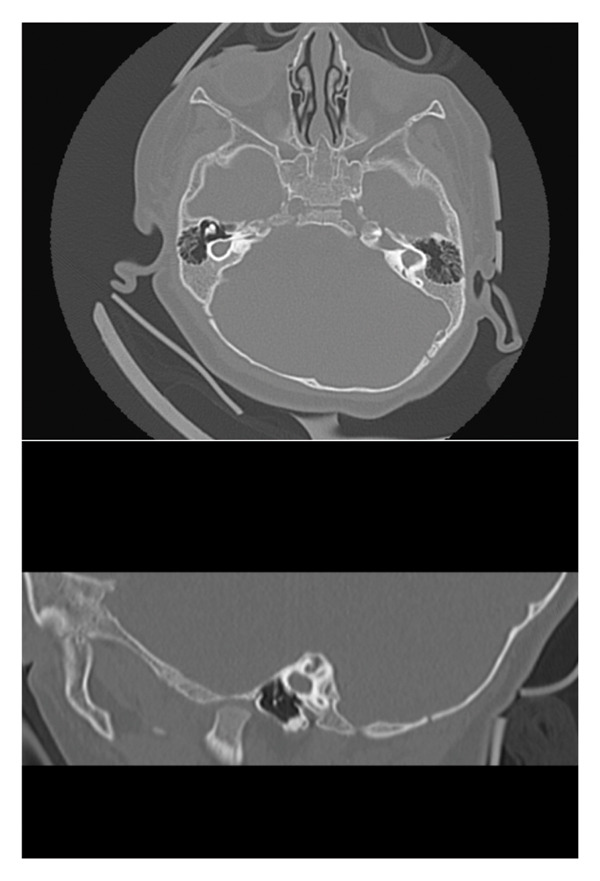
High‐resolution computed tomography (CT) demonstrating bilateral common cavity deformities. The selected axial and coronal reconstructions were chosen to allow simultaneous visualization of the malformation on both sides and show the absence of normal cochlear partitioning, with a single cystic cavity representing fused cochlear and vestibular structures, consistent with common cavity deformity.
